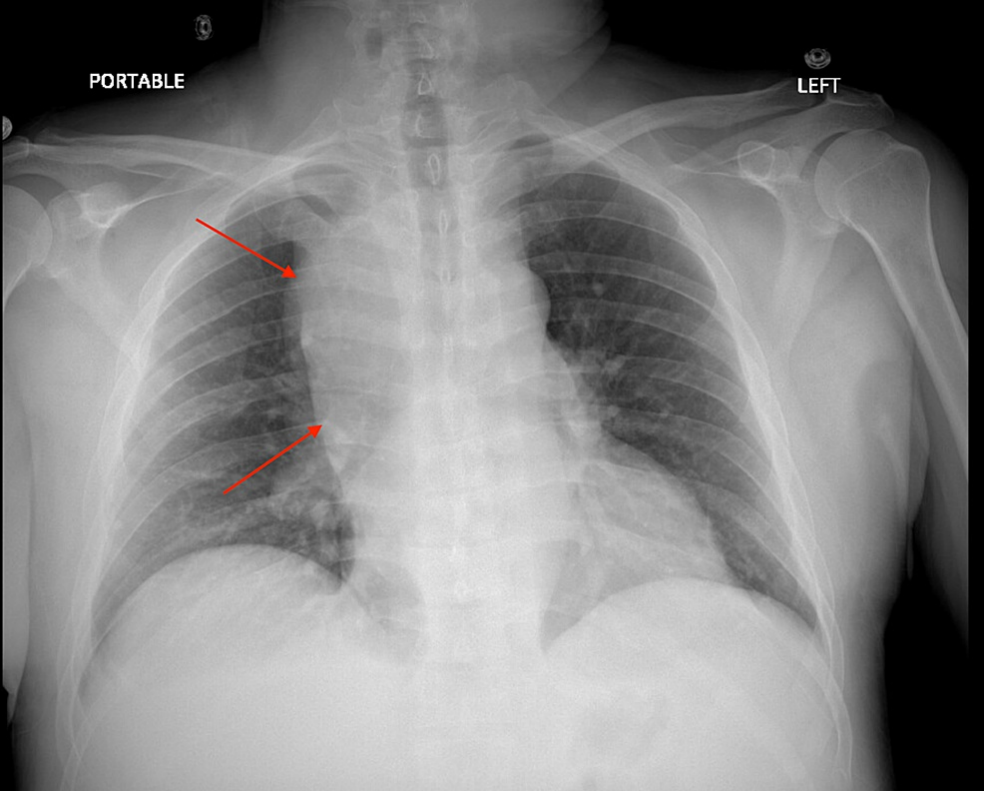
Chest X-Ray with large right-sided hilar mass
